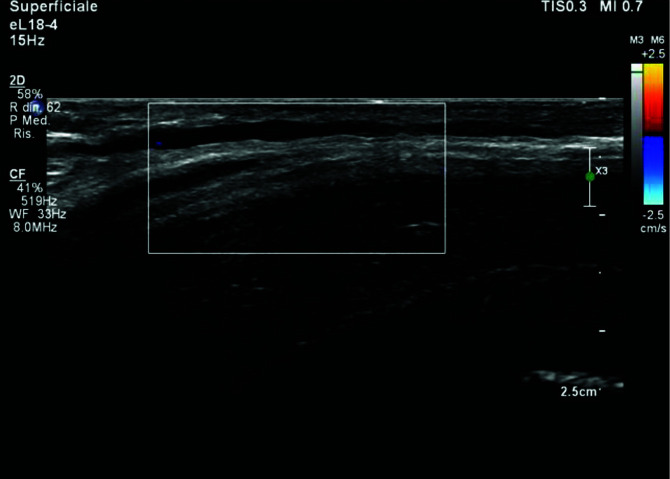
Superficial dorsal penile vein viewed with color doppler ultrasonography examination: no blood flow indicating thrombosis.
